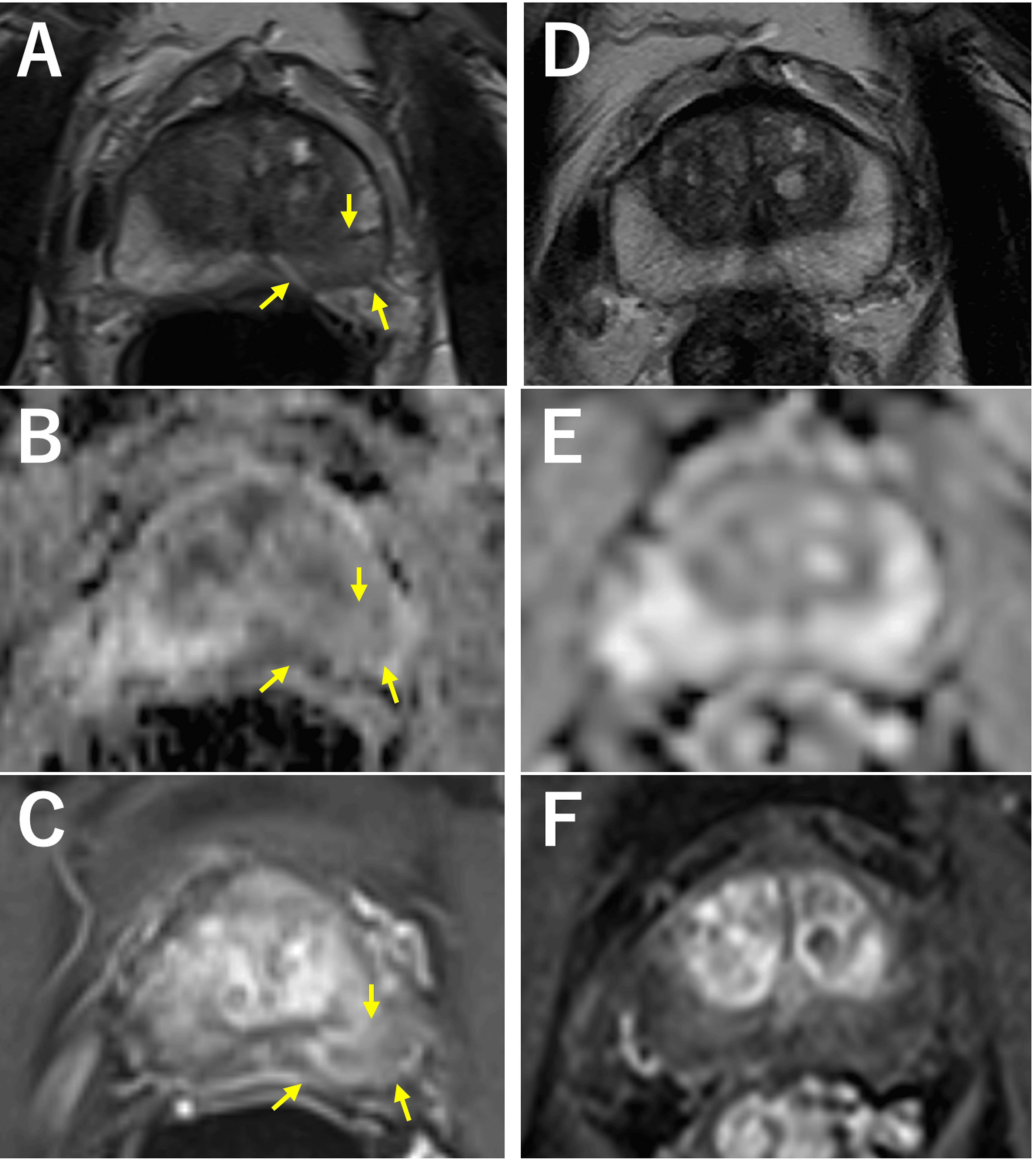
Multiparametric MRI of the prostate before (A-C) and after prednisolone therapy (D-F). Arrows indicate one of the inflammatory lesions exhibiting low-signal intensity on T2-weighted imaging (A) and apparent diffusion coefficient map (B) and slight enhancement on dynamic contrast enhanced imaging (C) at diagnosis. These inflammatory findings disappear after prednisolone therapy (D-F).
